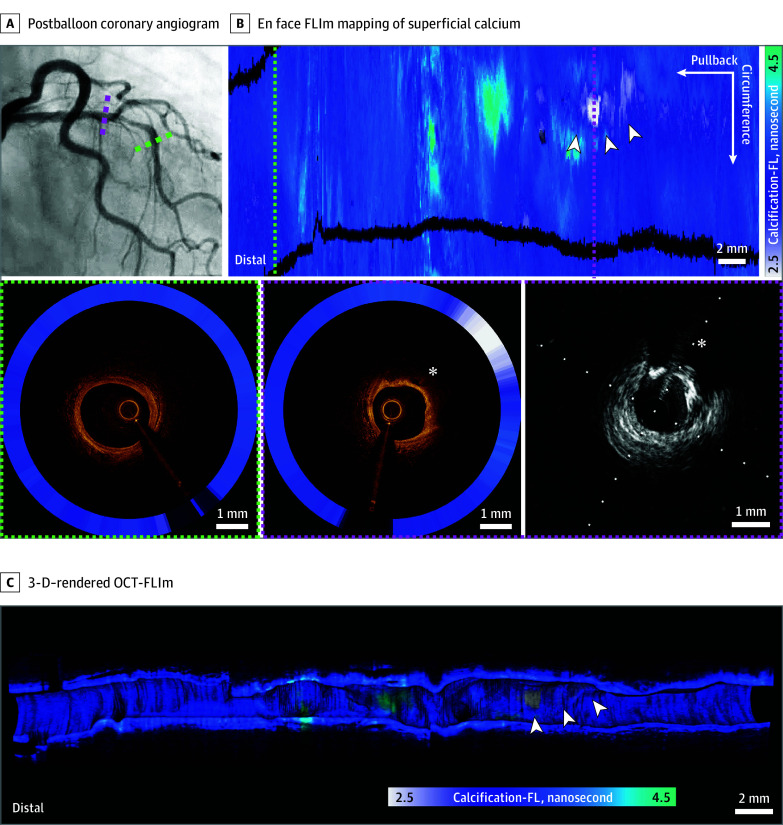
Optical Coherence Tomography and Fluorescence Lifetime Images of Superficial Calcification A, The postballoon coronary angiogram shows a calcified stenotic lesion in the proximal left anterior descending artery. B, The en face fluorescence lifetime imaging (FLIm) mapping of superficial calcium, identified by the 3 arrowheads, shows the lesion harboring various plaque compositions, including normal, fibrosis, and superficial calcium. The lower panel shows the cross-sectional optical coherence tomographic (OCT)–FLIm and intravascular ultrasound images of eccentric superficial calcification. The lower left panel shows a cross-sectional image of a normal arterial wall taken from the distal portion of image. The cross-sectional OCT-FLIm in the lower right panel shows a distinct molecular signature—defined as superficial calcification taken from area marked by the magenta dots in the upper panel. The asterisk in the lower right panel shows superficial calcification. C, Represents a 3-dimentional (3-D) rendering of the compositional signature of superficial calcium as indicated by the white arrowheads. The calcification-FL (fluorescence lifetime) ranged from 2.5 to 4.5 nanoseconds.
